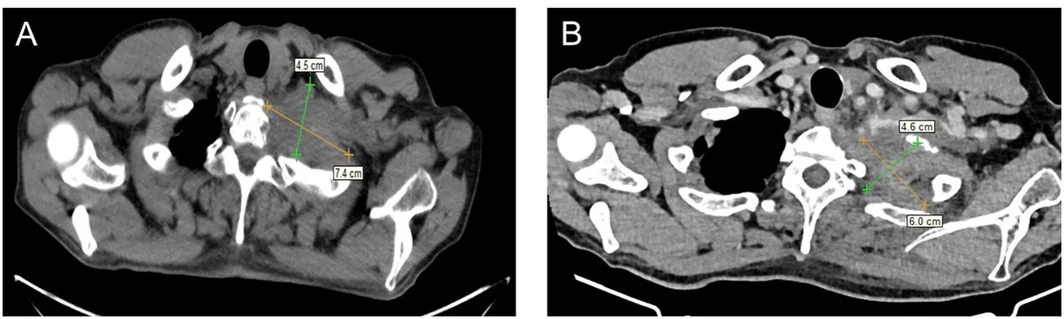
CT scans of the primary tumor lesion in the S1/2 of the left lung at immune checkpoint inhibition: (A) after three infusions of pembrolizumab (74 × 45 mm) and (B) the best response after 18 infusions of pembrolizumab (60 × 46 mm).
